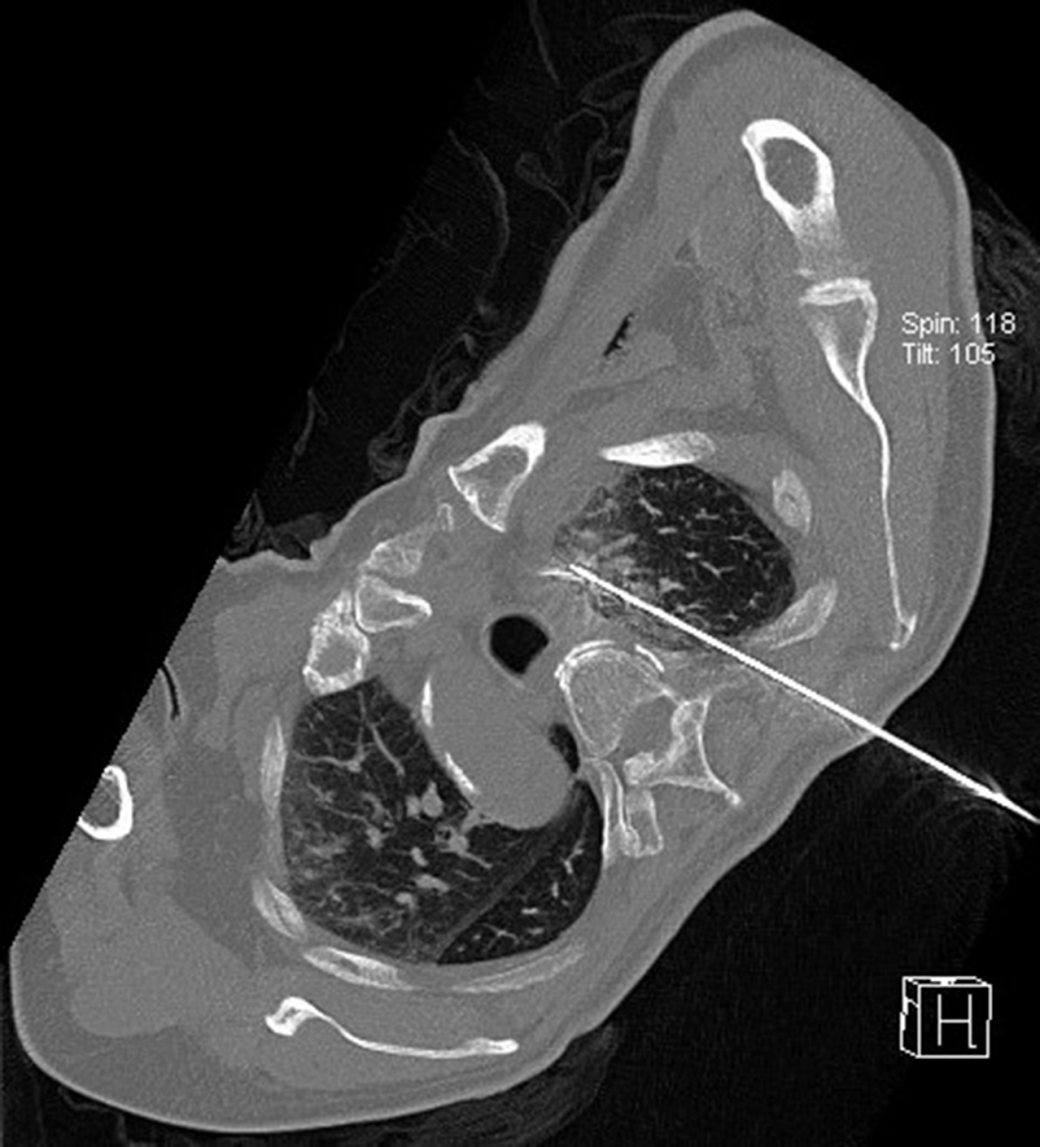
Intraoperative scan: the Reconstructed CT image.
